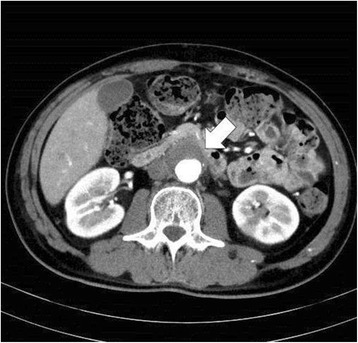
Second preoperative CT scan, seven days after the first CT scan. Axial view showing rapid expansion of the sacciform infrarenal abdominal aortic aneurysm (white arrow)
